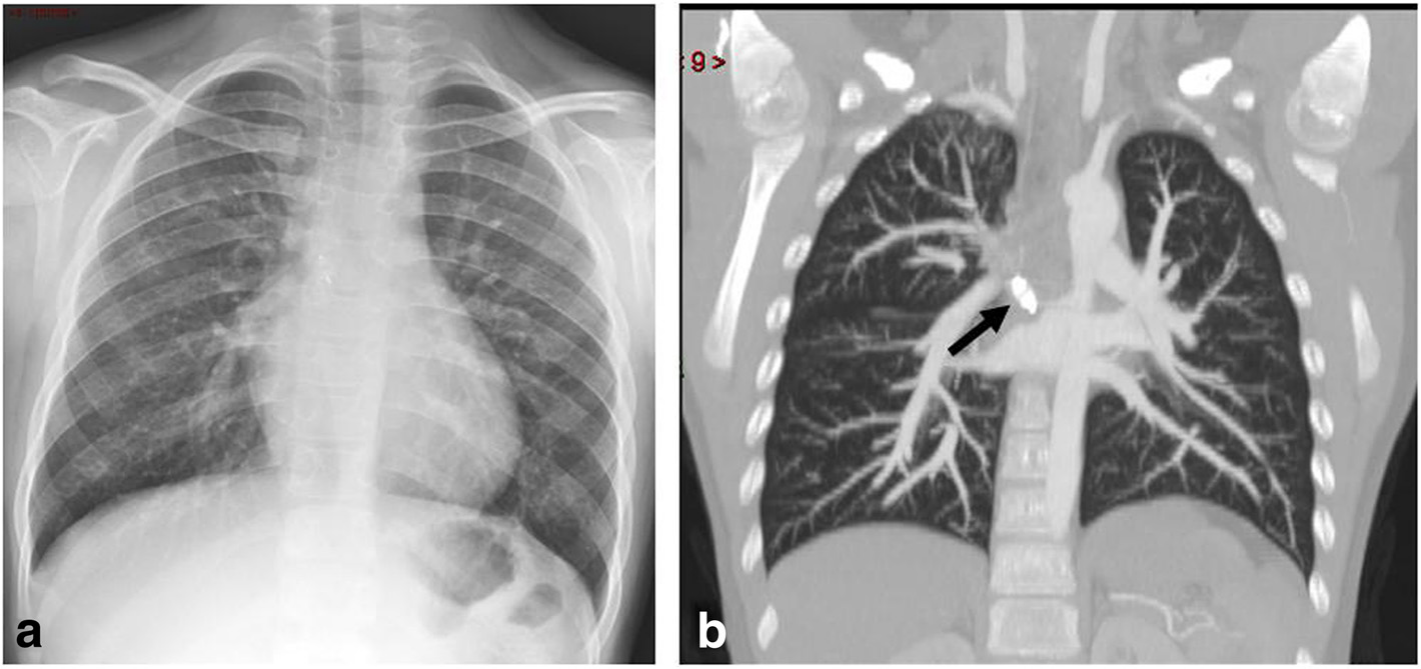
Chest radiogram (Panel a) and coronal multiplanar reconstruction (Panel b) of contrast-enhanced multidetector computed tomography showing normalization of the vascular pattern of the right lung (at 9-months follow-up). Vascular plug (*arrow*)
